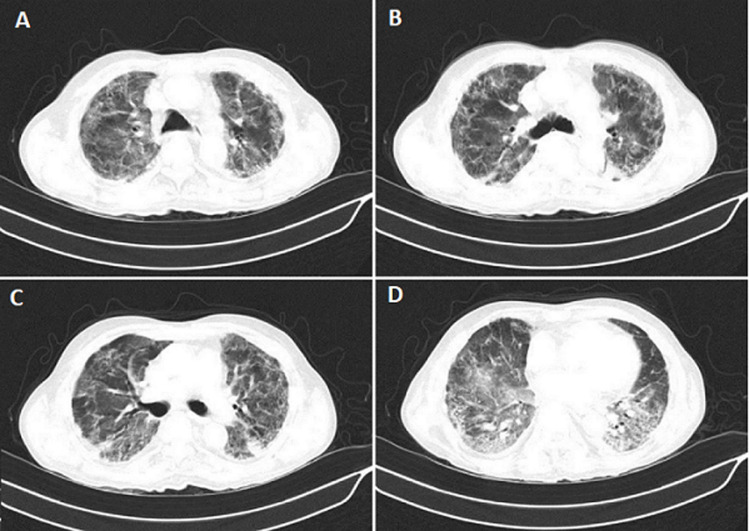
A) HRCT report showing fibrosis over the right lung; B) fibrosis over the left lung; C) bilateral consolidation; D) patchy ground glass opacity
